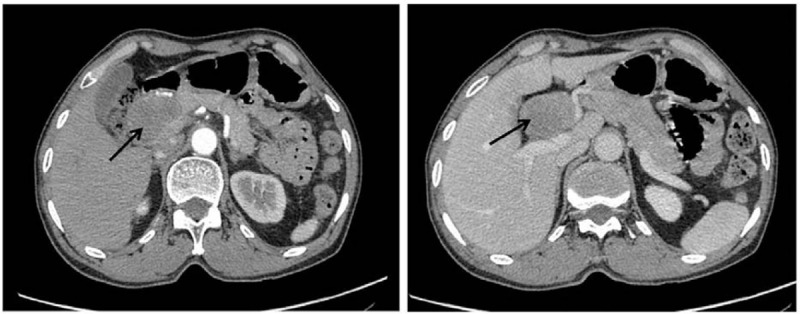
CT scans showing a 5 × 3.5 × 3 cm uniform density mass in the space between the porta hepatis and the stomach (arrow). CT = computed tomography.
